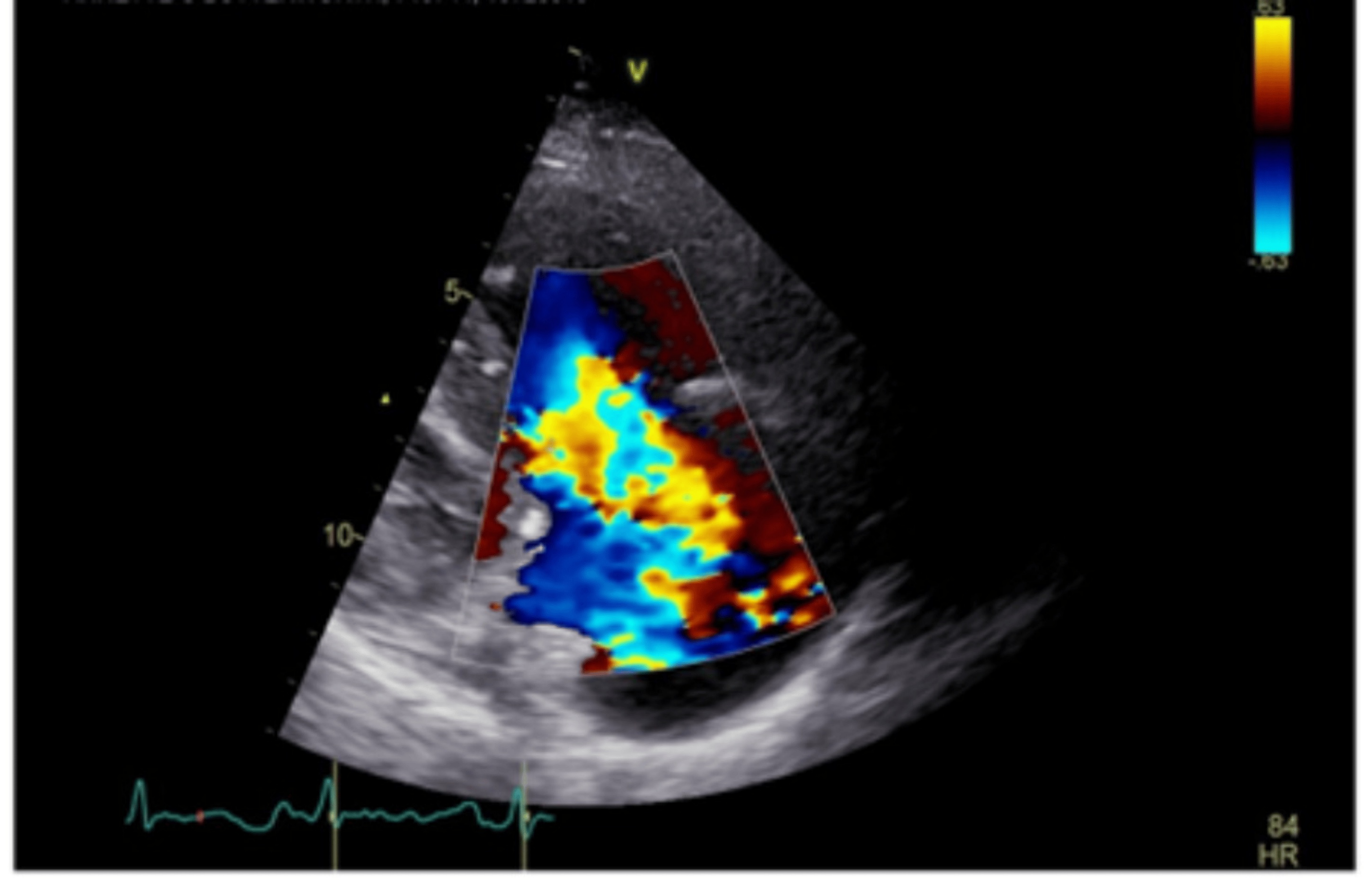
Severe tricuspid regurgitation on echocardiogram.
